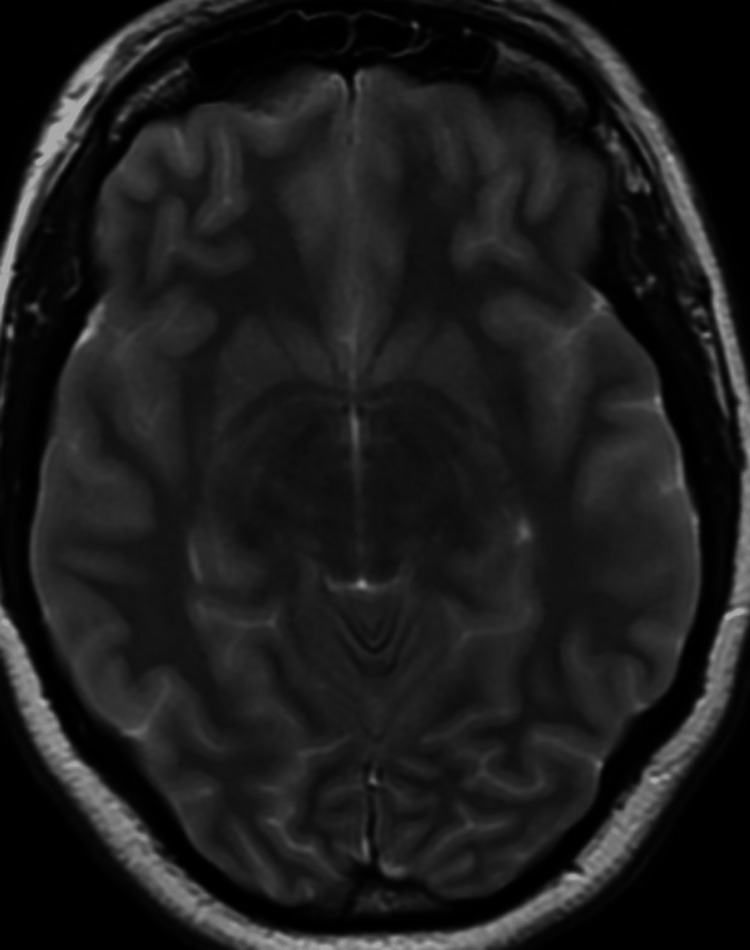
Magnetic resonance fast relaxation fast spin echo T2-weighted images of the brain on day three of admission Magnetic resonance imaging of the brain showing diffuse fast relaxation fast spin echo T2-weighted (FRFSE T2) hyperintensities throughout the cortex, subcortical white matter, deep gray matter with effacement of the cortical sulci, along with diffusion hyperintensity diffusely suggestive of severe adult hypoxic-ischemic injury.
